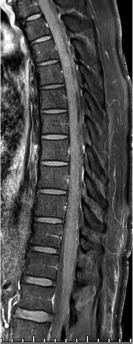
Sagittal post-contrast fat-saturated *T*1 weighted image MRI shows no evidence of abnormal enhancement in the affected segments of the cord.
